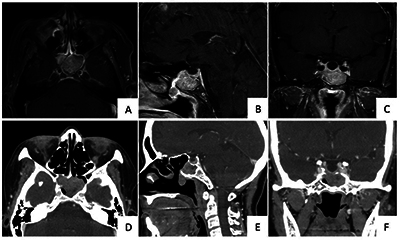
Atypical location of olfactory neuroblastoma in a 63-year-old woman presenting with epistaxis for 5 days. Contrast-enhanced T1-weighted imaging with fat suppression (A-C) and contrast-enhanced computed tomography (D-F) showed an enhanced mass within both sphenoid sinuses, slightly invading the sellar turcica.
